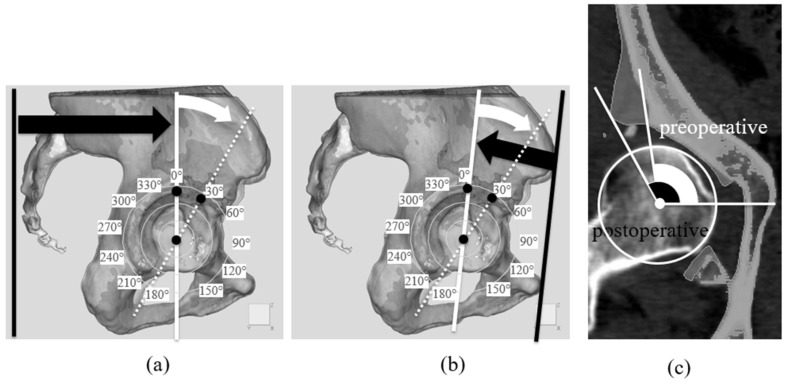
Evaluation method for the Acetabular Sector Angle (ASA). (a) The method for creating radial sections is shown. Using the preoperative and postoperative 3D models, radial sections were created by rotating 15° around an axis passing through the center of the femoral head. The position of the acetabular rim apex was defined as 0°, with the opposite acetabular rim at 180°, anterior at 90°, and posterior at 270°. Angles were defined clockwise for the right hip and counterclockwise for the left hip to unify the notation for both hips. The black line indicates the functional pelvic plane (FPP), defined using the inferior boundary of the axial CT image and the CT scanner coordinate system, which provides a standardized pelvic reference orientation for three-dimensional analysis, whereas the white line represents the reconstructed reference plane shifted to the 0° position to make radial section planes (Black arrow indicates the shift direction), and the white dotted line shows the plane for evaluation at the defined 30° position (white arrow indicates the rotational direction) as one example. (b) Using a similar method, the anterior pelvic plane (APP) was set as another reference plane. The black line represents the reference plane, whereas the white line represents the plane moved to 0°, and the dotted line represents the plane at the defined 30° position for evaluation. The black and white arrows serve the same purpose as in (a). (c) The ASA measurement method is shown. In the radial section, the angle between the center of the femoral head and the acetabular rim was defined as the ASA, and comparisons were made between preoperative (white) and postoperative (black) ASA.
